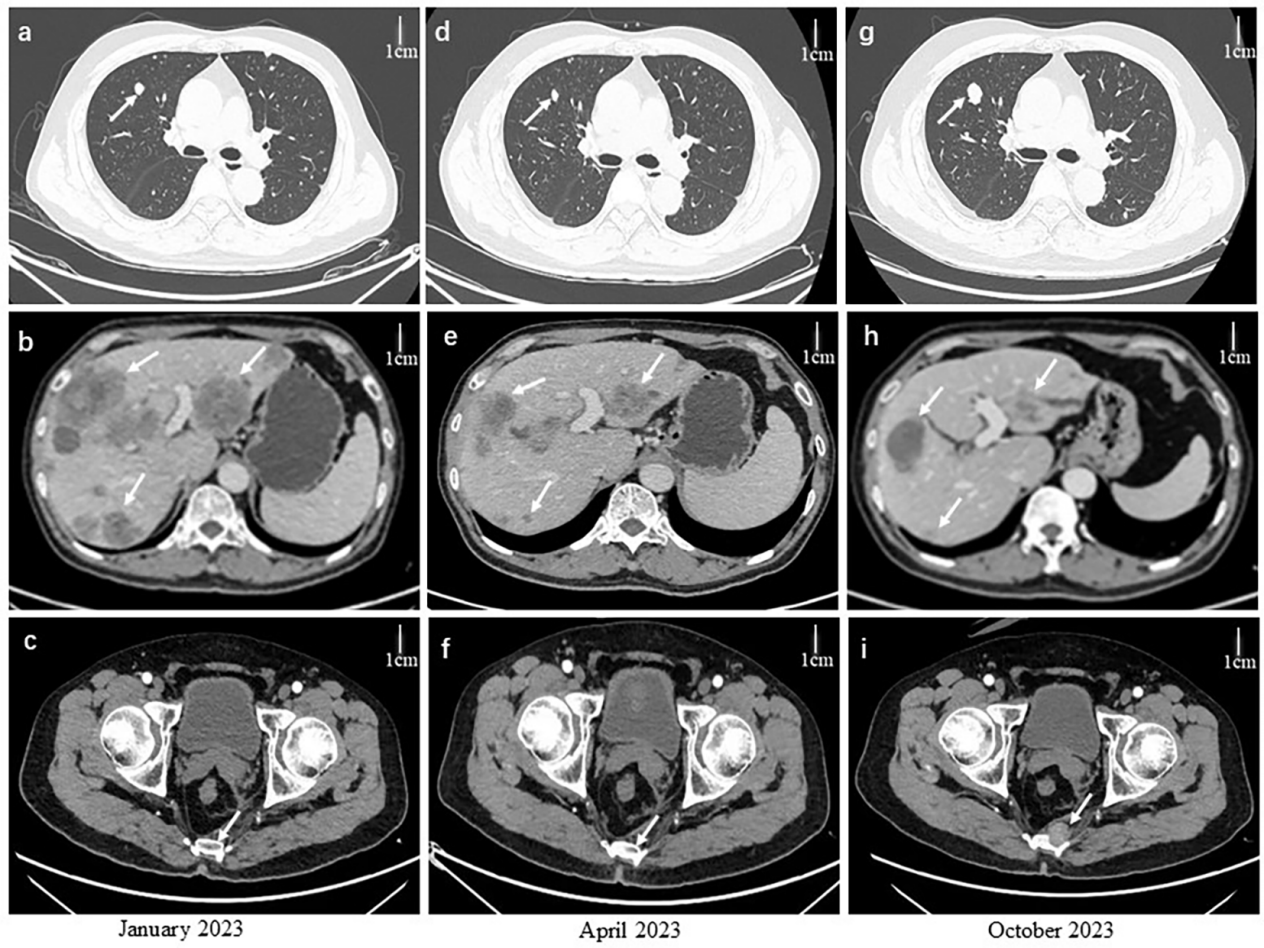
Arrows indicate tumor location. CT in January 2023 (after first line therapy) showed progression (a–c). April 2023 scans showed significant regression (d–f). October 2023 scans showed progression after trastuzumab plus pertuzumab with mXELIRI (g–i). Scale bars represent one centimeter.
